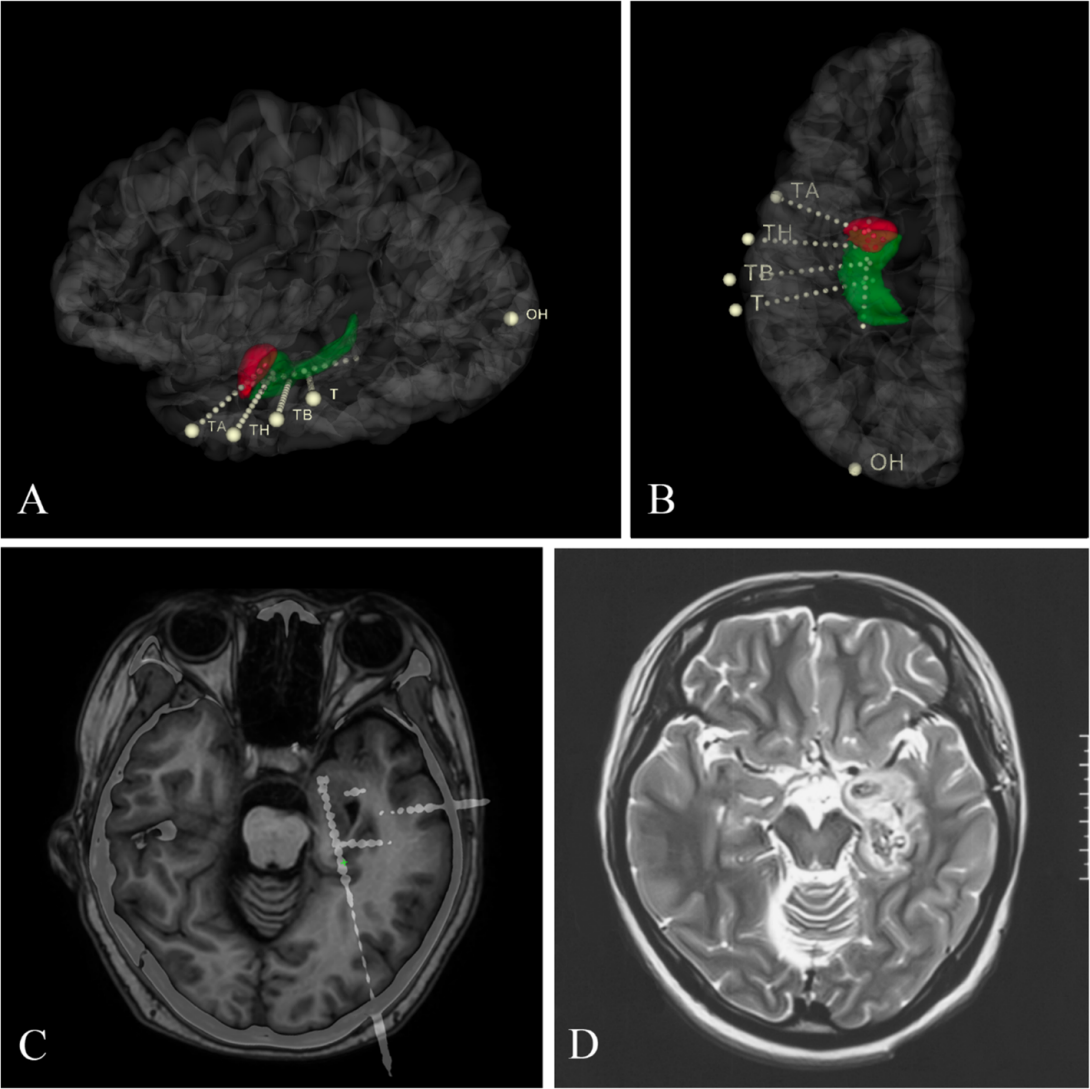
A, B The distribution of SEEG electrodes via three-dimensional reconstruction and the relationship between electrodes and the mesial temporal area. OH, T, TH, and TB were implanted in the hippocampus. TA was implanted in the amygdala. C The combination of preoperative T1-weighted images and postoperative CT images. The relative anatomical position of the SEEG electrodes and brain structure can be observed. D Postoperative T2-weighted MRIs obtained within 1 week of SEEG-guided RF-TC
